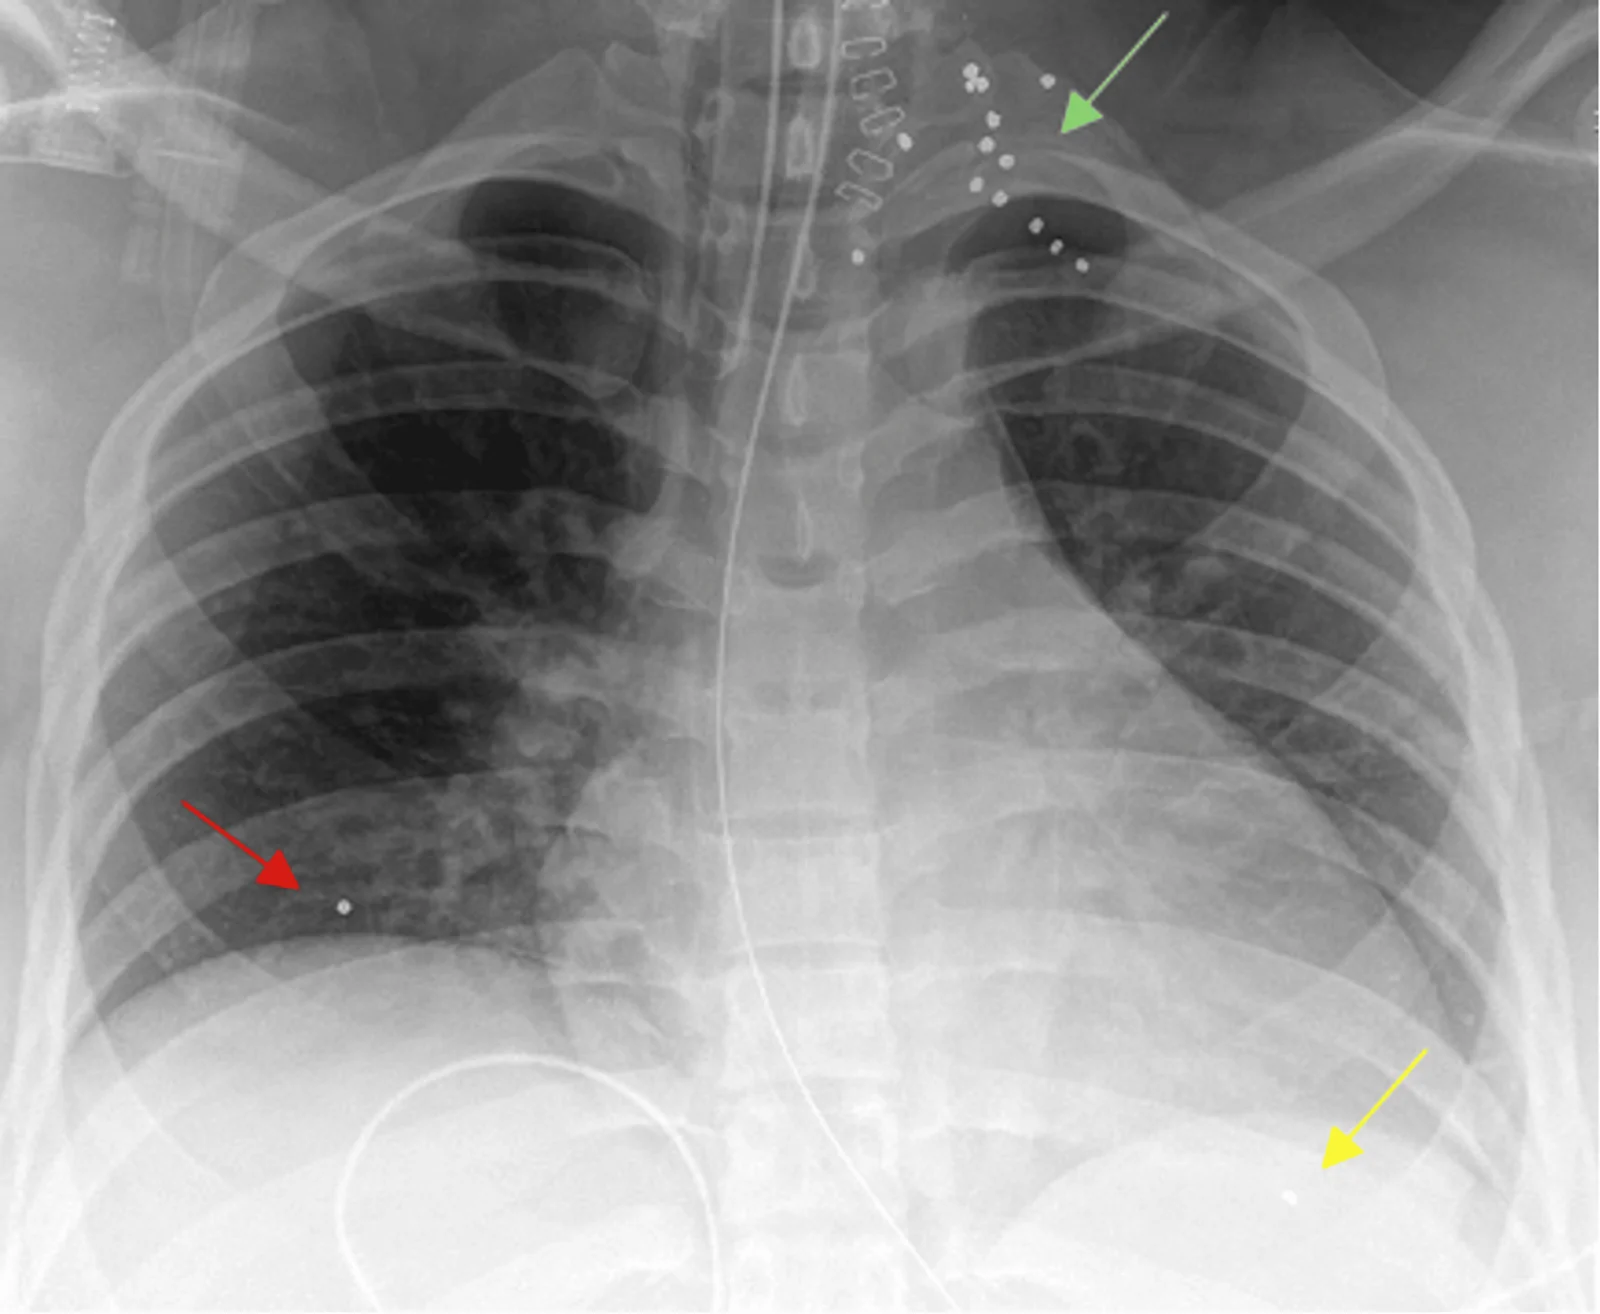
Postoperative chest X-ray showing stable locations of the pellets within the neck (green arrow), apex of the heart (yellow arrow), and right lung periphery (red arrow).
